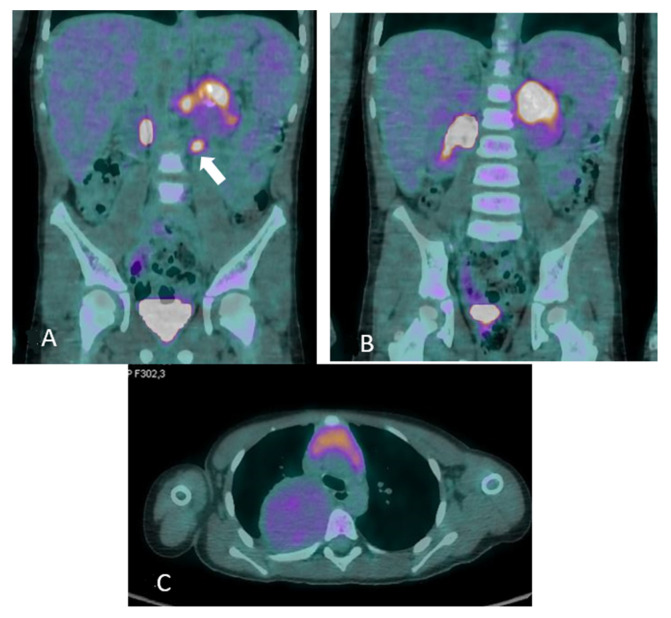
^18^F-fluorodeoxyglucose/computed tomography (^18^F-FDG PET/CT) at one year from diagnosis. The patient was in good general clinical condition and the disease was stable; however, there were critical issues that were determined by the inability to rule out a nodular component within the already biopsied thoracic mass or the unstudied abdominal mass (see Figure 7). In order to characterize the metabolic activity of the mass, a ^18^F-FDG PET/CT scan was performed and showed FDG uptake in the left paravertebral mass (A) (maximum standardized uptake value -SUVmax-6.0), in the para-aortic lymph nodes (B) (maximum size 1.2 cm, SUVmax 5.8) and in the thoracic mass (C) (SUVmax2.3); no tracer uptake was detected in other sites. Tumor markers remained stable and within normal limits at all evaluations. The radiological and metabolic features assessed by ^18^F-FDG PET have not allowed the nature of the lesions to be clearly defined; in particular, with the available radiological investigations, the differentiation of benign and malignant NTs is quite controversial so far. Nevertheless, the different FDG uptake of abdominal lesions with lymph node involvements suggests a nodular component. In addition, the biopsy performed at onset may have been unrepresentative of the whole tumor, leading to late misdiagnosis with significant clinical fallout.
